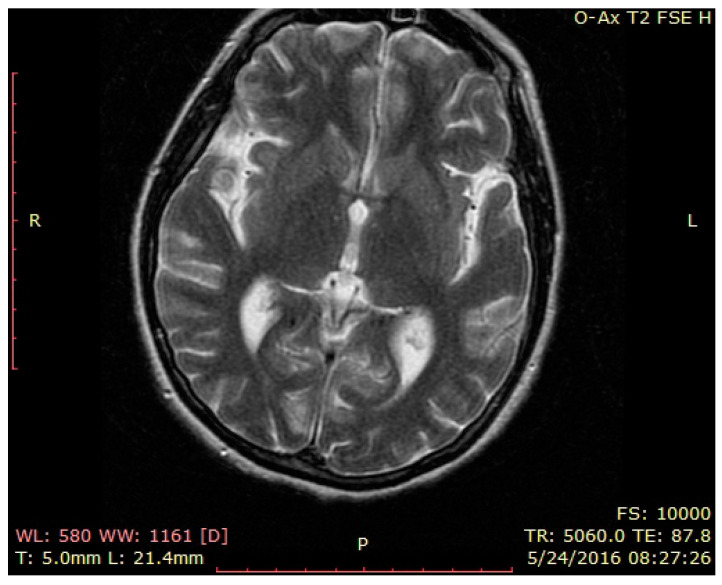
Brain MRI—normal findings (T2 weighted brain scan), axial insular image 1.
